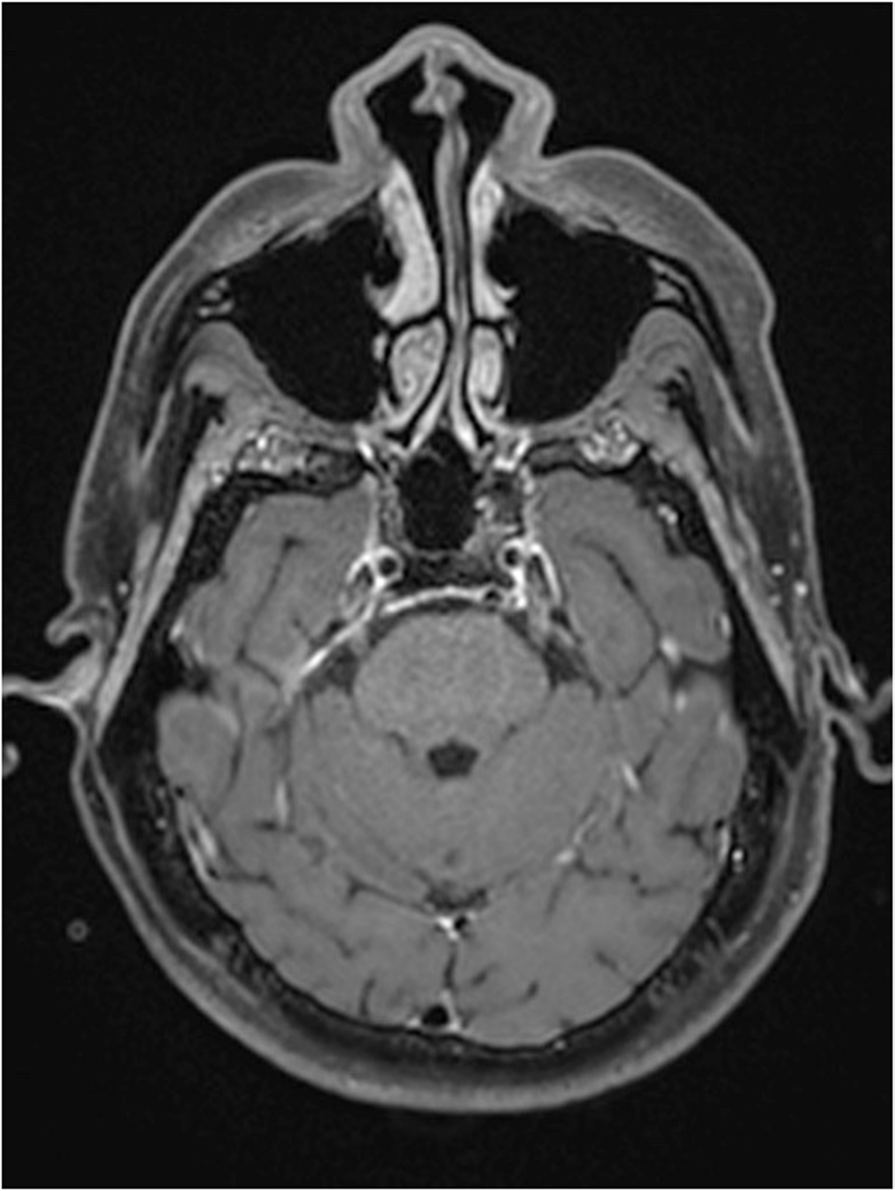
Contrast MRI brain shows mild contrast enhancement of trigeminal nerves bilaterally
